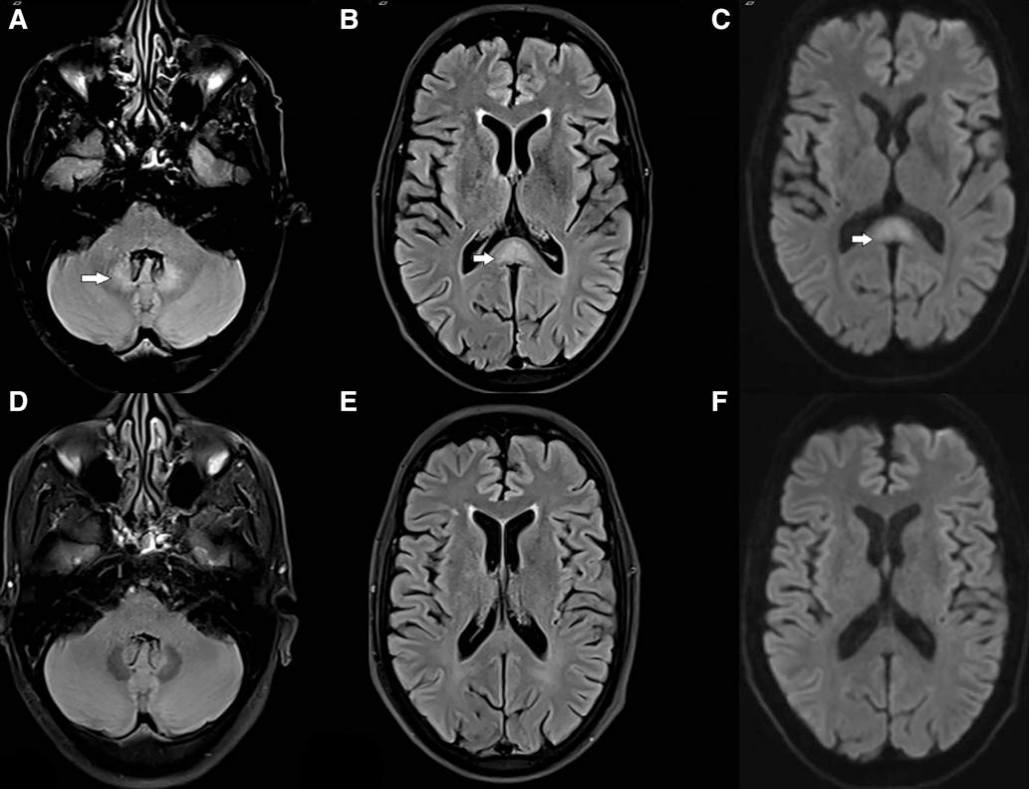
Axial fluid-attenuated inversion recovery (FLAIR) (A and B) images show symmetrical FLAIR hyperintensities in bilateral dentate nuclei and splenium of corpus callosum with restriction on DWI in the splenium, (C) suggestive of metronidazole toxicity (arrow marks). Repeat imaging performed after 2 months revealed complete resolution of the lesions (D–F).
